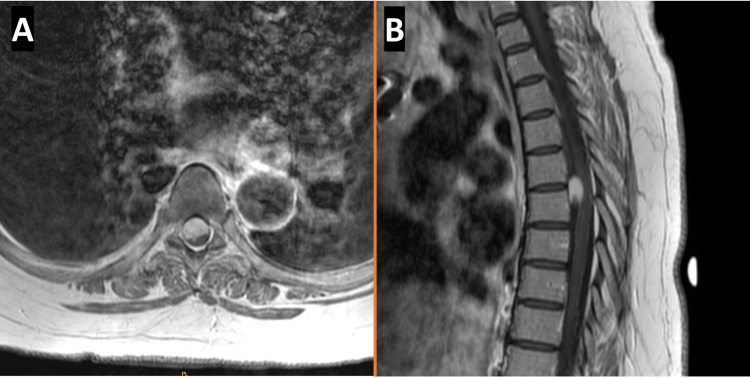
Preoperative T1-weighted post-contrast MRI with axial (A) and sagittal (B) views showing the IERC. IERC: Intradural extramedullary renal cell carcinoma.
